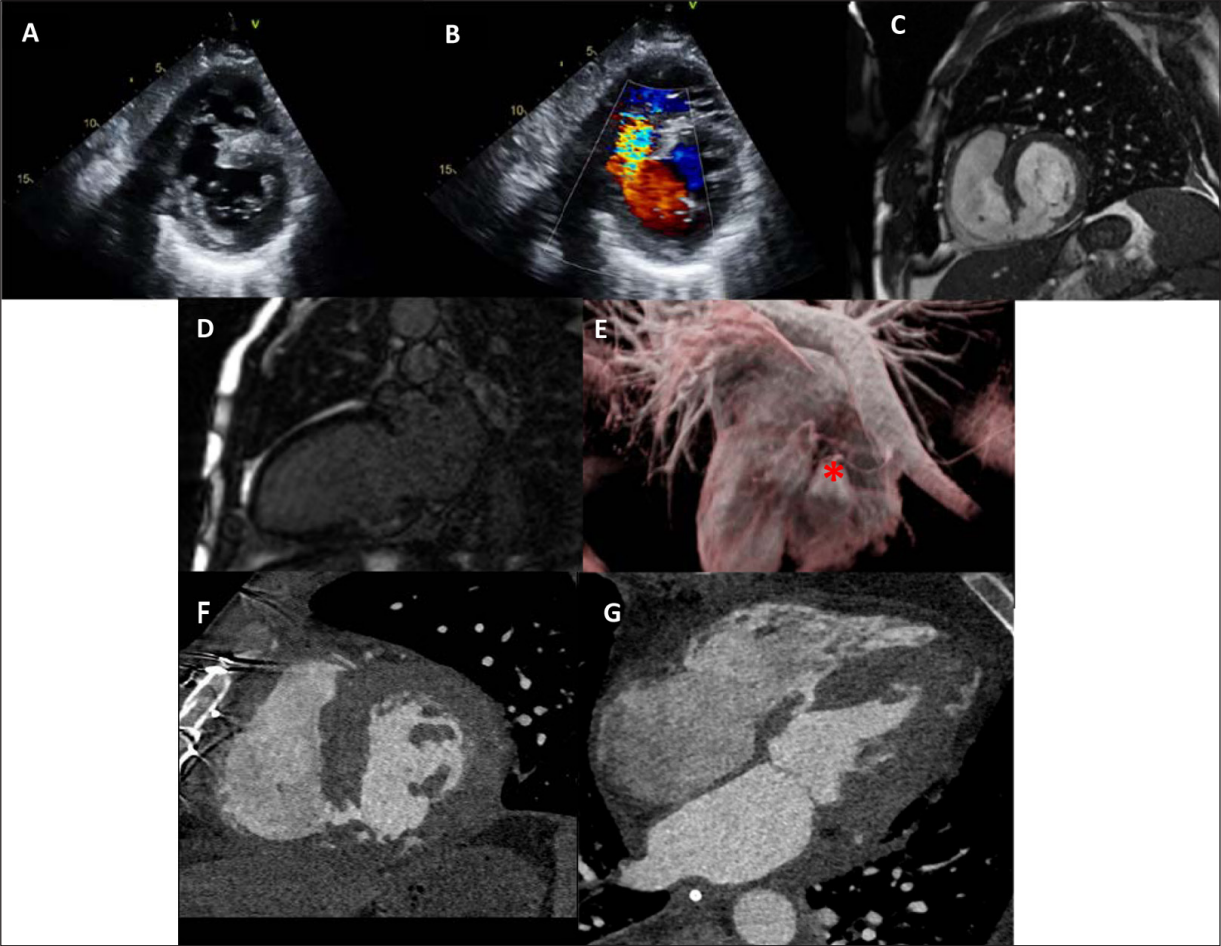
(A) Transthoracic echocardiogram of basal short-axis view showing inferoseptal ventricular septal rupture (VSR) and (B) color Doppler of inferoseptal VSR with left-to-right shunt. (C) Cardiac magnetic resonance imaging (CMR) of basal short-axis view showing inferoseptal VSR and (D) 2-chamber view of nonviable basal inferior wall. (E) Cardiac magnetic resonance of 3-dimensional reconstruction showing inferior aspect of the heart with inferior wall aneurysm (red asterisk). (F) Computed tomography of residual ventricular septal defect showing short-axis view and (G) 4-chamber view.
